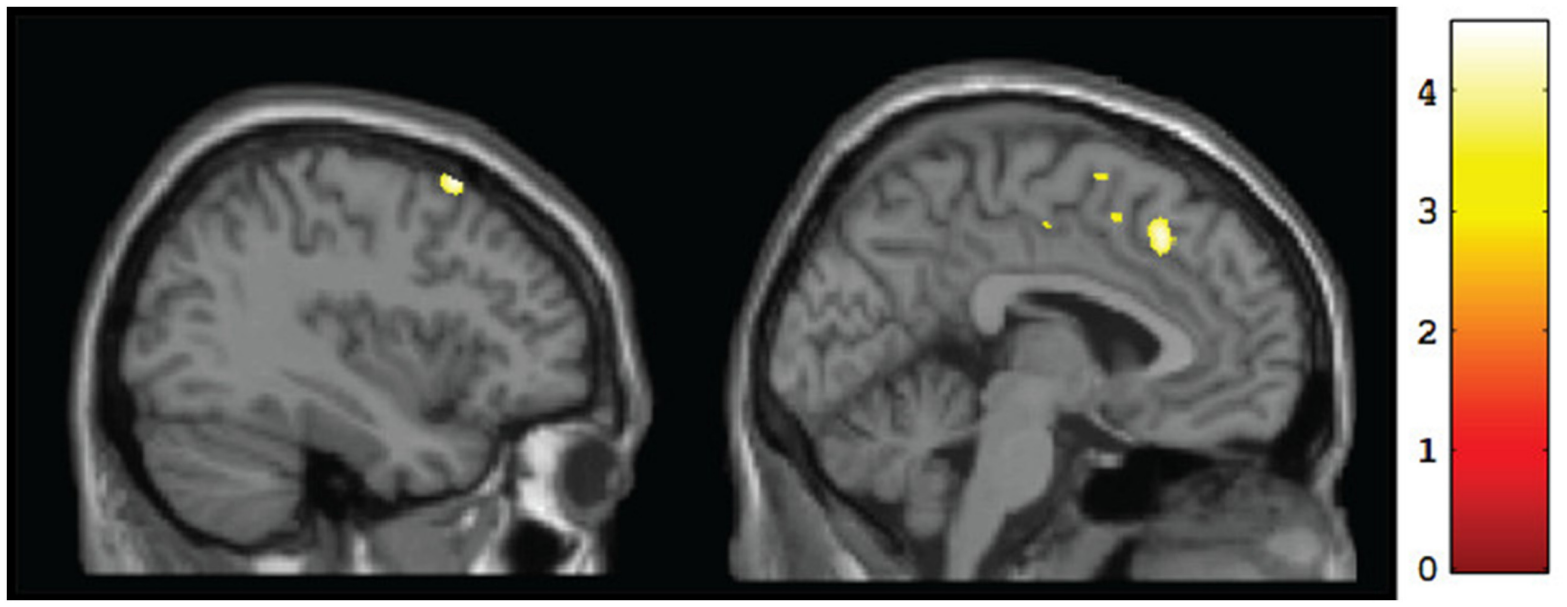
Sagittal views of regions showing significant (*p* <0.001, uncorrected) differences in gray matter volume at baseline for CT vs. FASD (TX and DTC combined): left superior frontal gyrus (BA8) [−37, 17, 60] (left) and left medial frontal gyrus (BA8) [−3, 25, 44] (right).
